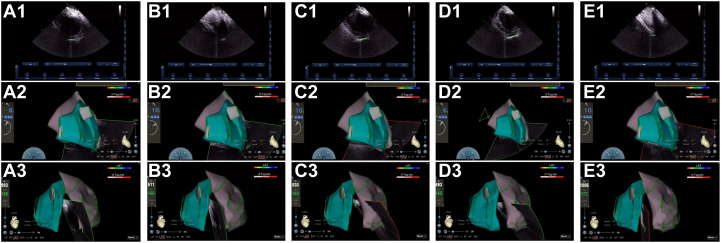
The Process of ICE Delineating the Pericardial Boundaries (A) Near-septal side of the left ventricular apex; (B) left ventricular apex; (C) left ventricular lateral wall; (D) left ventricular free wall; (E) left ventricular apex. 1) Intracavitary ultrasound plane; 2) right anterior oblique position; 3) left anterior oblique position. ICE = intracardiac echocardiography.3.The visualization of puncture needle: Connect the unipolar lead to the distal end of the puncture needle and integrate it with the CARTO system for real-time visualization of the needle tip. A vascular-specific puncture needle (16 GA 5.25 IN/1.7 × 133 mm) was used in this case (Figure 7A). The tail end of the needle is connected to the “a” end of a custom-made connection cable (Figures 7B to 7D), which then connects the “b” and “c” ends to the PINBOX of the CARTO system. This visualization enabled accurate tracking of the puncture needle's trajectory as it advanced.
